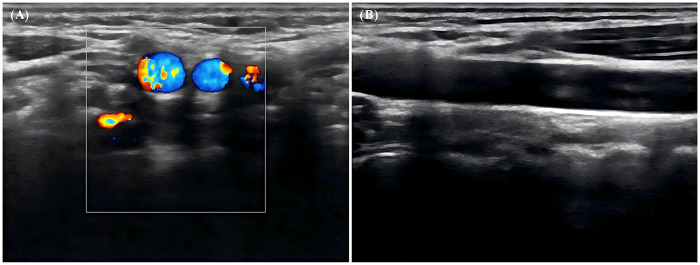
Last available postoperative imaging follow-up by ultrasonography on July 26, 2018. (A) Transverse color Doppler image of the postoperative left carotid bifurcation region. (B) Longitudinal grayscale image of the postoperative operative site. No definite recurrent mass or obvious vascular abnormality is identified on this examination; no further imaging surveillance was available thereafter.
